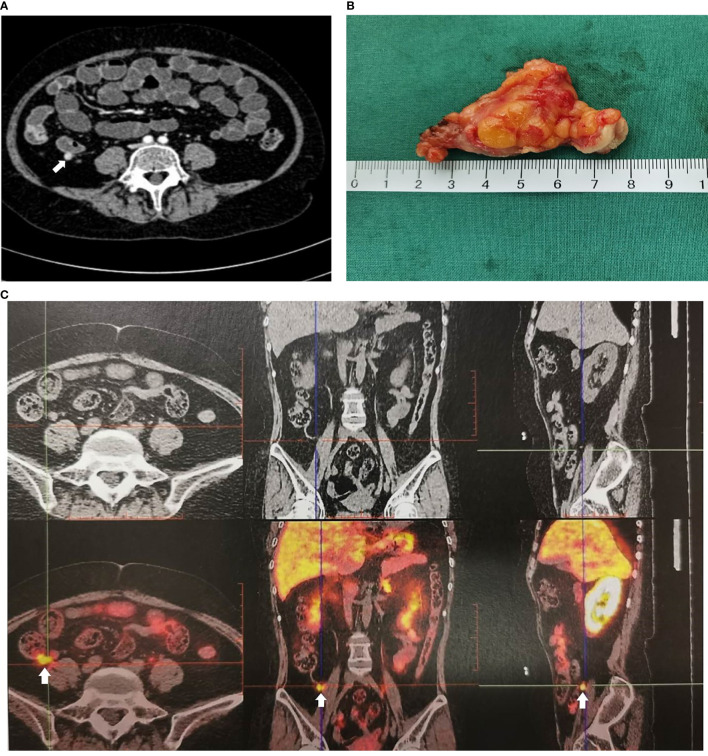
Imaging examination of appendiceal mass. (A) Appendiceal mass showed by contrast enhanced CT reconstruction of the small intestine. (B) Appendiceal mass. (C) Appendiceal mass showed by ^68^Ga-DOTATATE-PET-CT.
